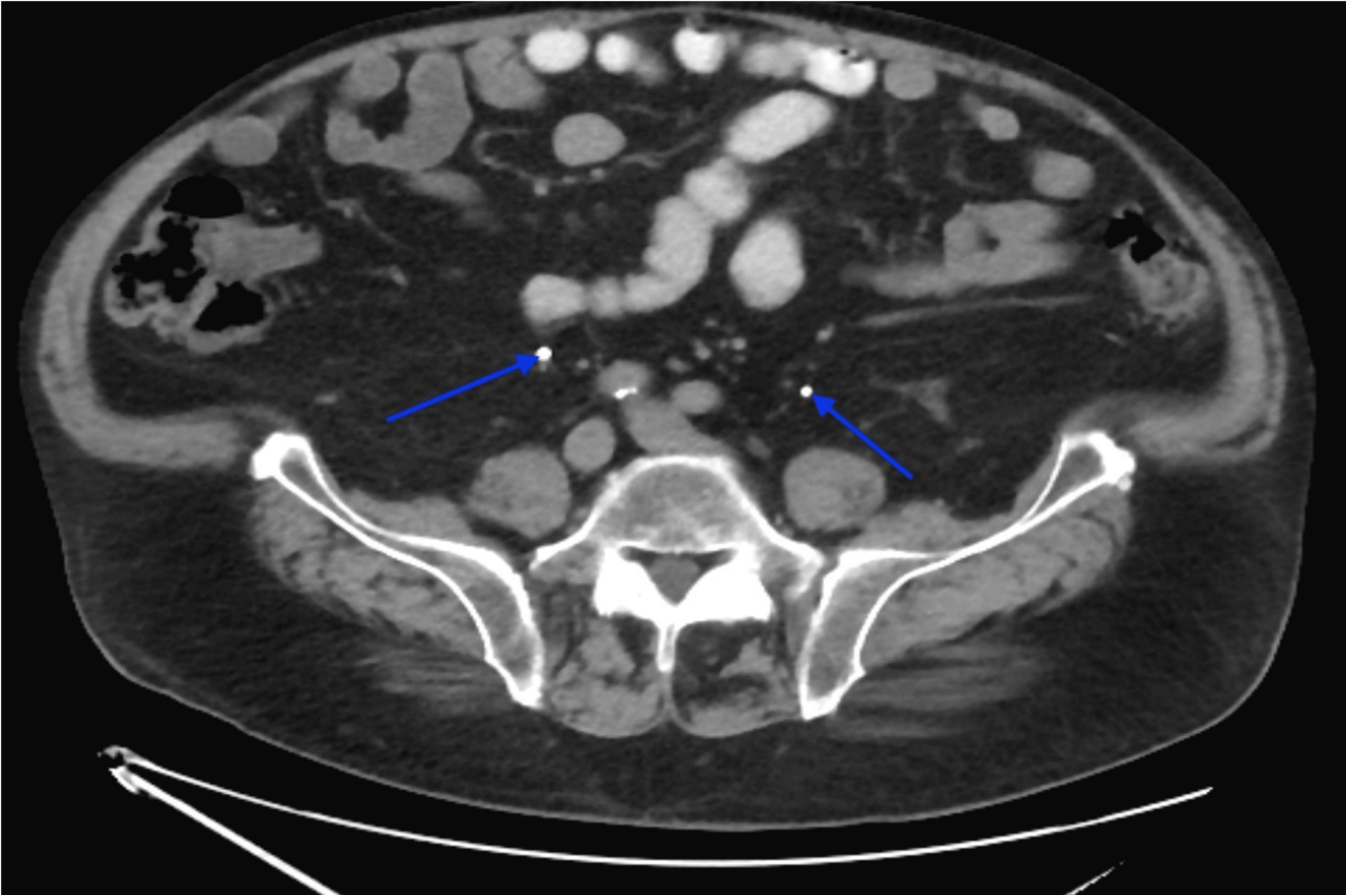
CT with contrast demonstrating significant anterior descent of patient's ureters (blue arrows) bilaterally within the abdomen.
